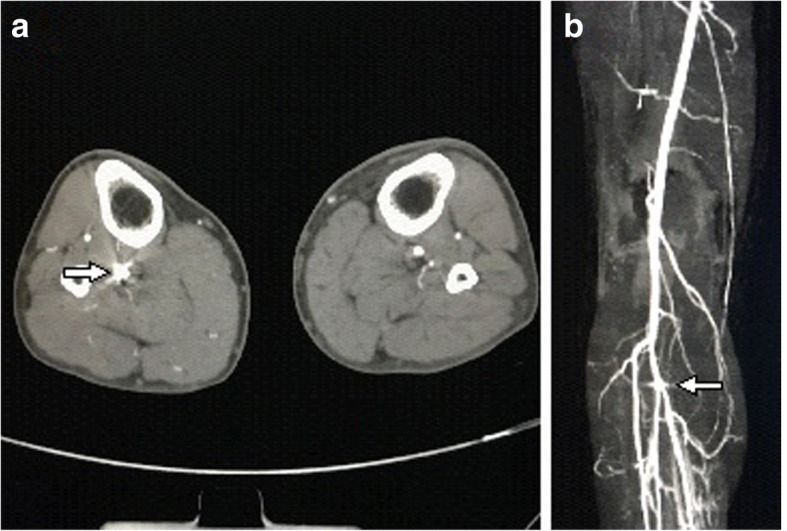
a Computed tomography (CT) angiogram axial view of the lower limbs. The arrow points to an artifact occluding the right TPT. b CT angiogram coronal view of the right lower limb. The arrow points to an artifact occluding the right TPT
